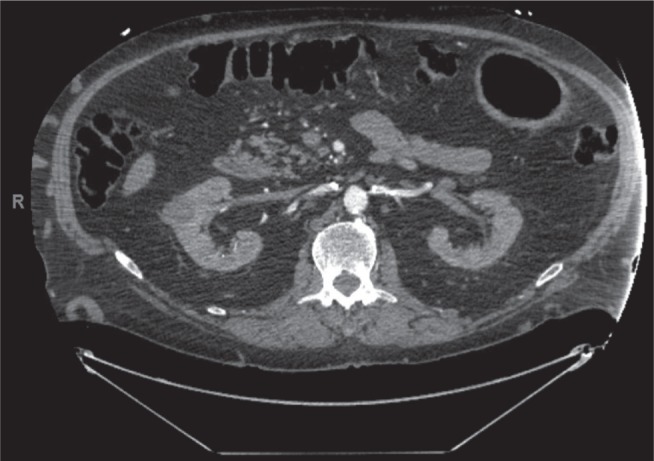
Abdominal CT with contrast showing the abdominal aorta with renal artery branches. Renal veins are also seen, but the IVC is absent.
